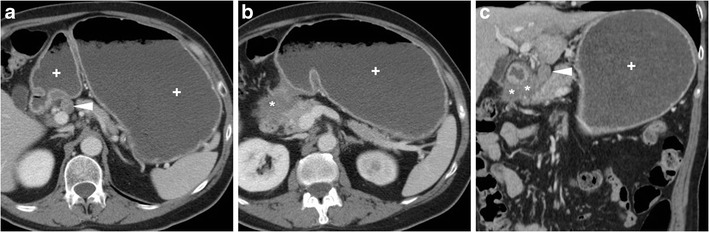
In a 77-year-old male, obstructing duodenal carcinoma appeared at CT as an eccentric, poorly enhancing tissue (*) abutting the proximal duodenum, causing upstream gastric fluid-filled dilatation (+). Note adjacent round-shaped solid lymphadenopathy (arrowheads)
